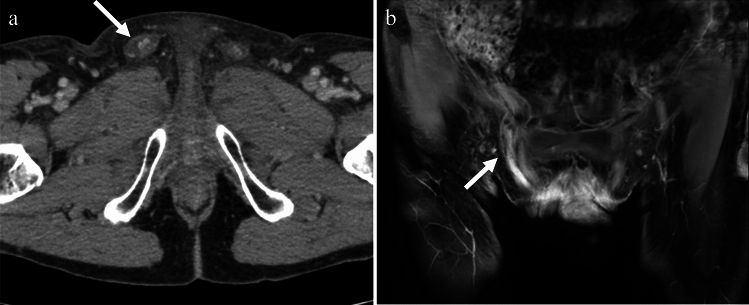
Man in his 60 s with right groin tenderness with unknown causative pathogen. a Contrast-enhanced CT reveals swelling in the right inguinal canal (arrow). b Coronal fat-saturated contrast-enhanced T1-weighted image reveals conspicuous hyperenhancement of the right spermatic cord (arrow), suggestive of vasitis
